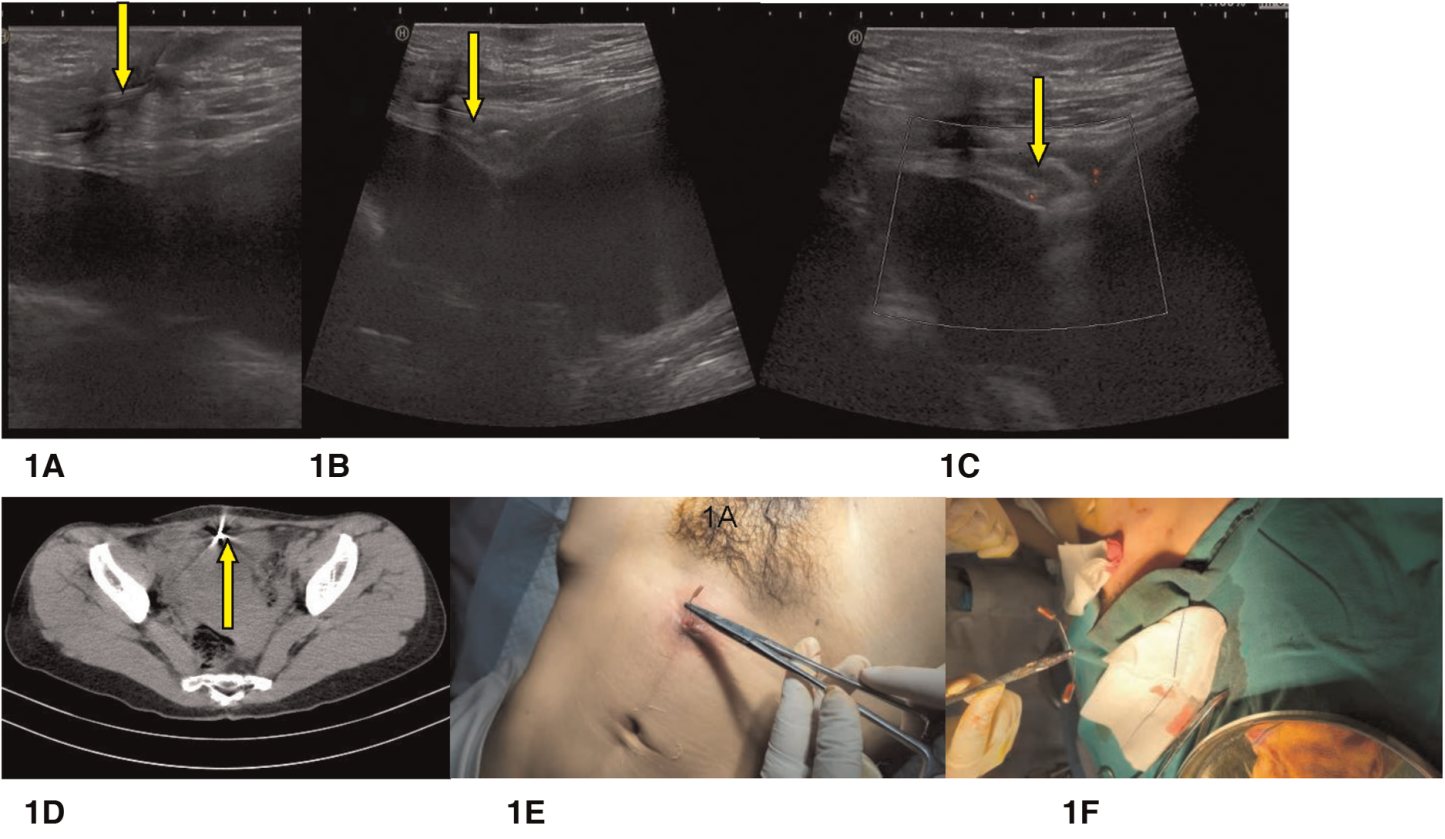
A 34-year-old woman presented with an abscess of the abdominal wall around the IUD, which had been discharging pus for 10 days. Yellow arrows indicate the IUD. (A) In this longitudinal section of the lower abdominal wall, linear hyperechoes (IUD) can be seen, folding the rectus abdominis through the center of the cesarean scar. (B) In this cross-section of the inferior abdominal wall, linear hyperechoes (IUD) can be seen, clasping the straight muscle of the abdomen through the center of the cesarean scar. (C) In this cross-section of the lower abdominal wall, abscess echoes can be seen in front of the anterior wall of the bladder, and dotted blood flow signals can be seen surrounding them. (D) CT cross-section of the inferior abdomen. A V-shaped metal IUD can be seen on the lower abdominal wall. (E) Part of the IUD was removed from the lower abdominal wall during the operation. (F) The MCu-IUD was extracted from the inferior abdominal wall during the operation. IUD: intrauterine device.
